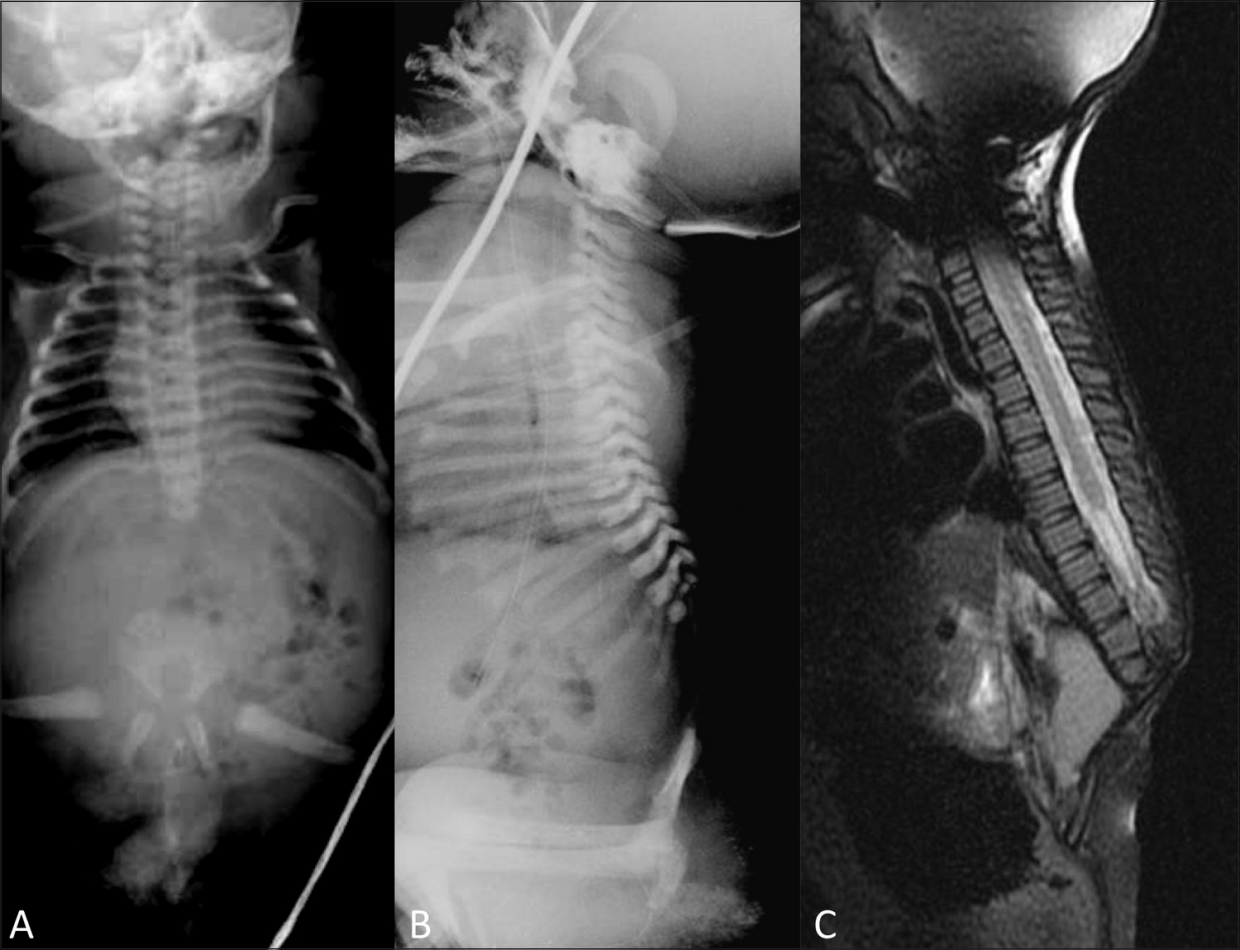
Caudal regression syndrome: Frontal (A) and lateral (B) radiographs of the spine show absence of the sacrum and the entire lumbar spine. The pelvis is very small and the pelvic bones are fused at the midline. Sagittal T2W MRI of the spine (C) shows severe caudal regression with complete absence of the lumbar spine and sacrum. The conus medullaris has a characteristic, abnormal, wedge-shaped (blunted) appearance
